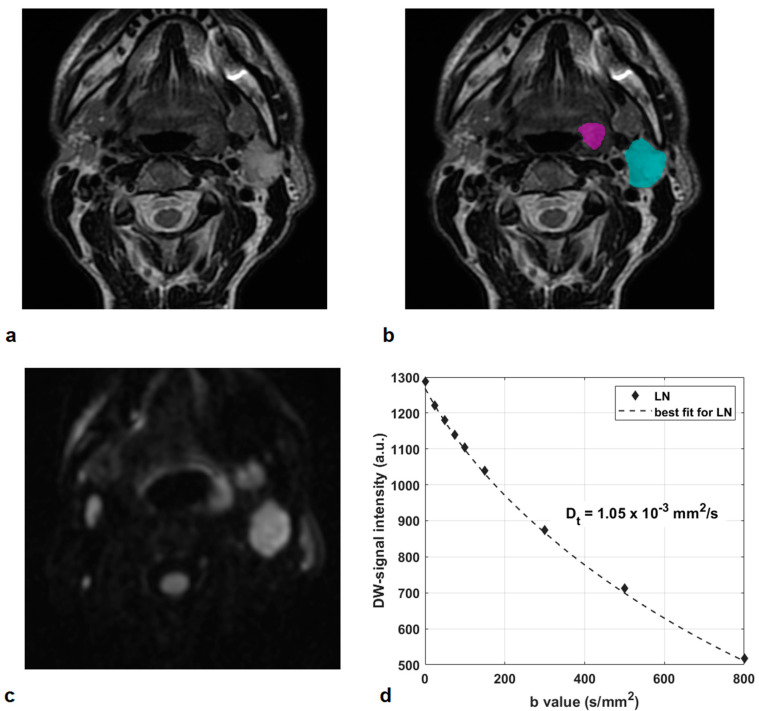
HPV-positive oropharyngeal squamous cell carcinoma of the tonsil in a 71-year-old man, non-smoker, and non-alcoholic (Patient 3): T2-w images (a,b) show the primary tumor (contour in magenta) and a large lymph node (contour in cyan). The corresponding DWI at b = 800 s/mm^2^ (c) and DW-signal intensity curve at increasing b values in the LN (d). A restricted water molecule mobility was observed in the LN, with ADC and D_t_ values of 1.17 and 1.05 × 10^−3^ mm^2^/s, respectively.
